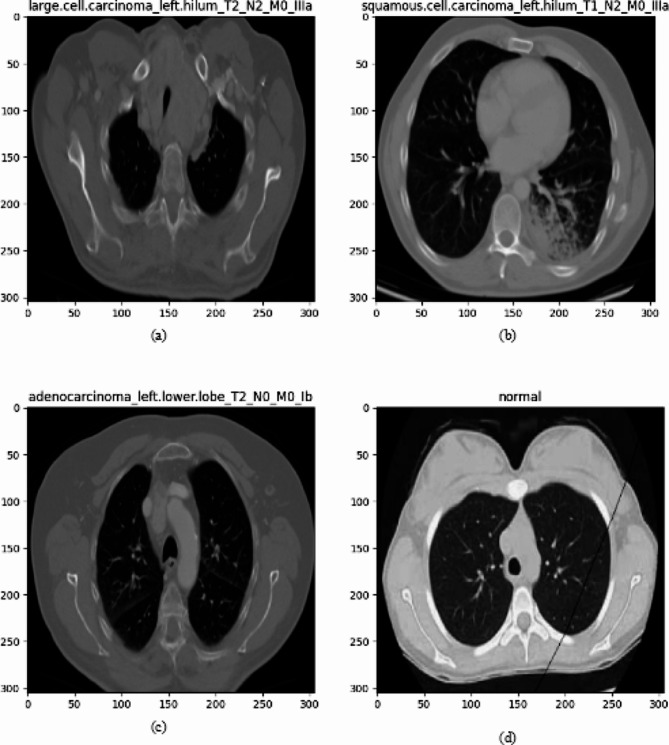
Sample images from chest CT imaging dataset (a) large cell, (b) squamous cell, (c) adenocarcinoma, and (d) normal
